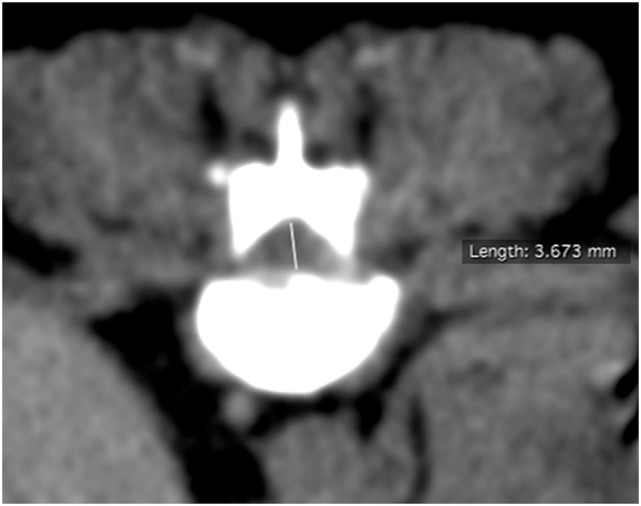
Preoperative transverse computed tomography image at site of maximal compression with measurement of minimal cross-sectional dimension.
